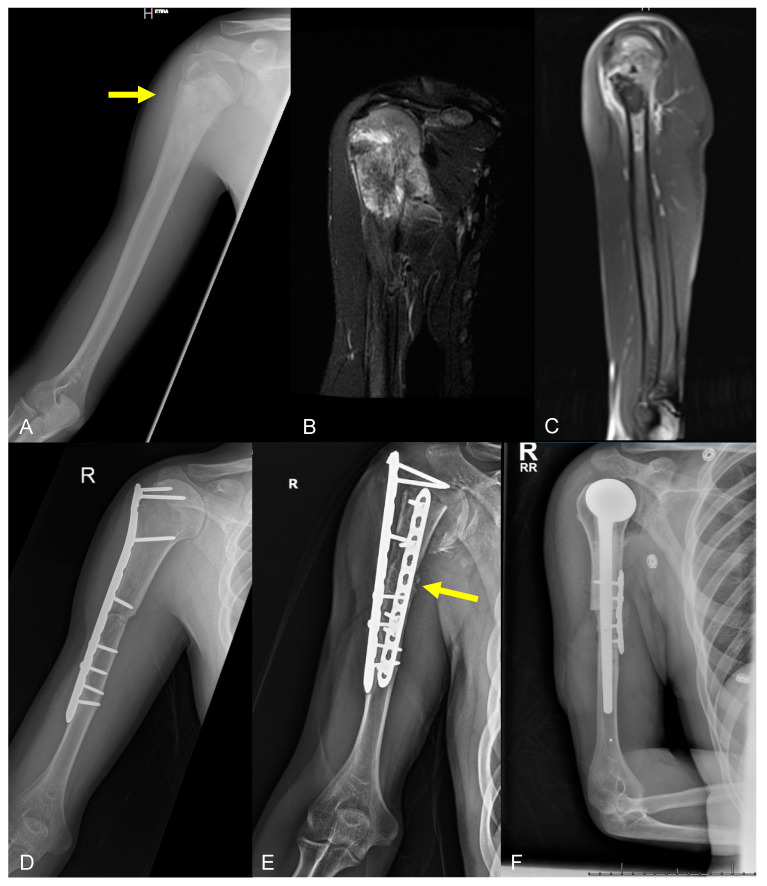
Imaging of an 18-year-old male, with history of high-grade osteosarcoma of the proximal humerus diagnosed at age 14 who received neoadjuvant chemotherapy, wide resection and osteoarticular allograft reconstruction, and revision surgery for nonunion 2 years after initial reconstruction (type 2B failure), now presenting 4 years after initial reconstruction with functional pain, found to have allograft fracture (type 3B failure). Initial (A) radiograph and (B,C) MR images of the tumor at diagnosis. (D) Radiograph demonstrating initial proximal humerus allograft reconstruction after tumor resection. (E) Radiograph showing allograft fracture. (F) Post-operative radiograph after revision to alloprosthetic hemiarthroplasty.
